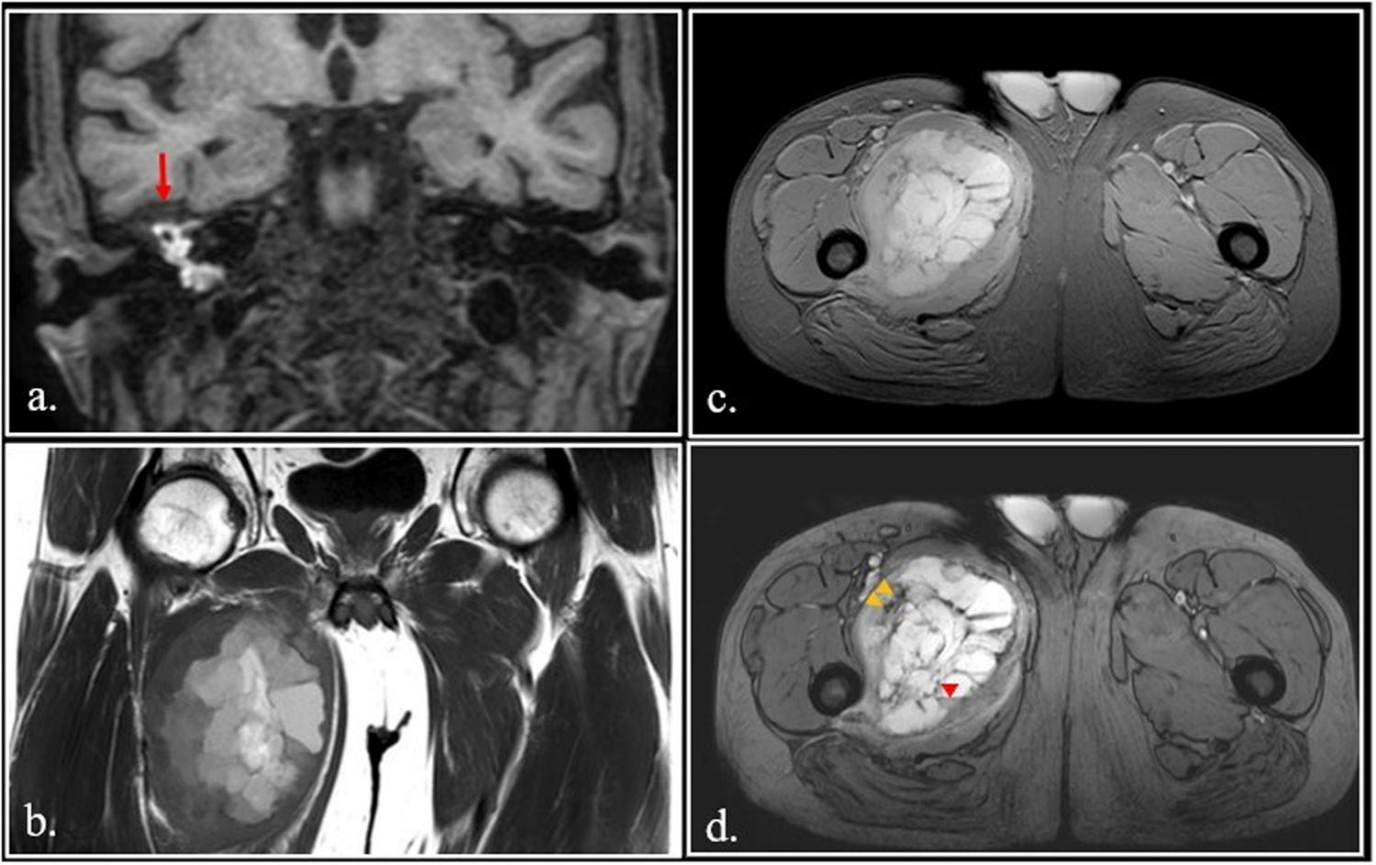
a Coronal FSE fat-sat T1-weighted image. Typical high signal cholesterol granuloma (arrow) of the right middle ear. b–d Multicystic telangiectatic soft tissue sarcoma of the abductor muscles of the left thigh. On Coronal FSE T1-weighted image (b), the cysts demonstrate different degree of hyperintensity due different concentration of T1 shortening substances. Dual Gradient-Echo (GE) T2*-weighted images obtained at 20 ms (c) and 40 ms (d) show fluid-fluid interfaces. At 40 ms (d), there is a blooming by susceptibility artifact at the fluid-fluid interfaces (red arrowhead) and in the solid component of the neoplasm (yellow arrowheads). The susceptibility phenomenon demonstrates that the hyperintensity of the lesion is due to methemoglobin in a hemorrhagic lesion
